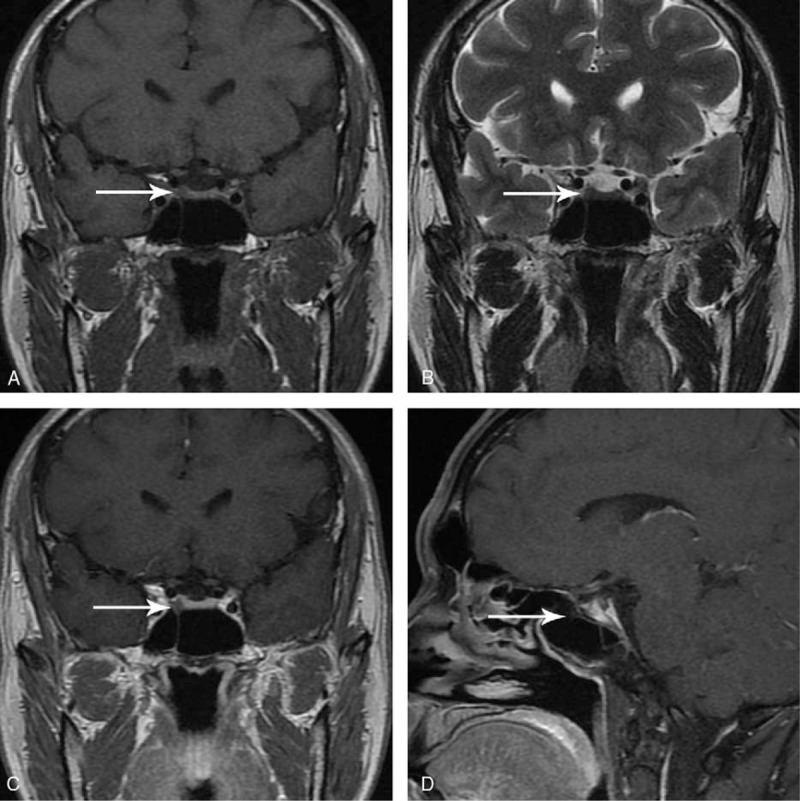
Preoperative MRI characteristics of the pituitary lesion: (A) coronal T1WI, (B) coronal T2WI, (C) coronal-enhanced T1WI, (D) sagittal-enhanced T1WI). MRI = magnetic resonance imaging, T1WI = T1-weighted image, T2WI = T2-weighted image.
